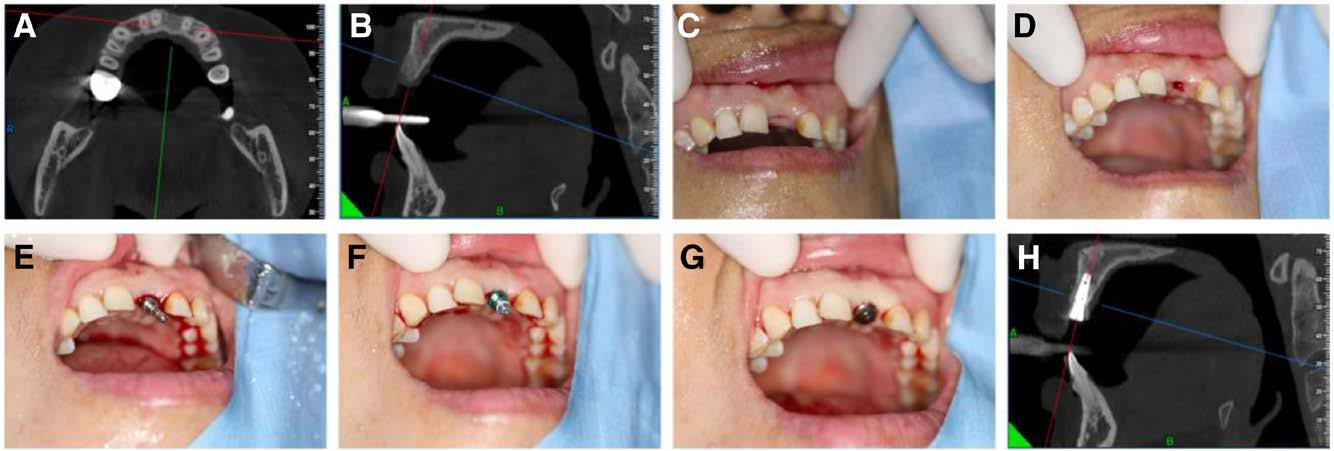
(A) Conical beam computed tomography (CBCT) shows preoperative bone integrity on cross section; (B) conical beam computed tomography (CBCT) showed preoperative bone integrity on sagittal plane; (C) a preoperation picture showing tooth loss in a front view; (D) touch-controlled minimally invasive flapless implant nest preparation; (E) detect the orientation of implant nest preparation; (F) the implant was inserted into the alveolar bone; (G) the implant is fitted with a healing abutment; (H) bone profile with an implant after the surgery.
